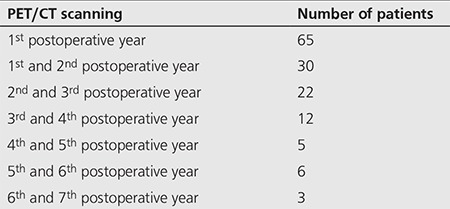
Patient distribution according to time of PET/CT scanning
